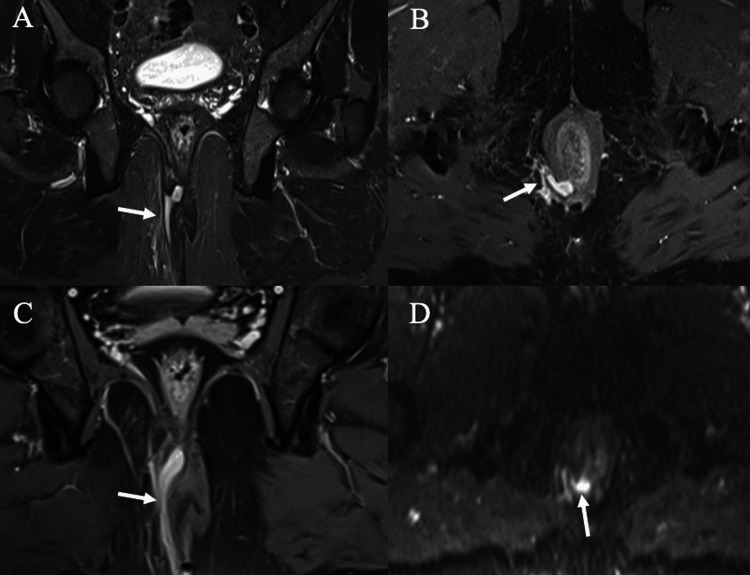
Grade 3 trans-sphincteric fistula. (A) Coronal STIR image showing right trans-sphincteric fistula. (B-C) Axial and coronal post-contrast-enhanced fat-suppressed T1 images, respectively, showing right trans-sphincteric fistula. (D) Axial DWI showing restricted diffusion on DWI. DWI: diffusion-weighted imaging, STIR: short tau inversion recovery
